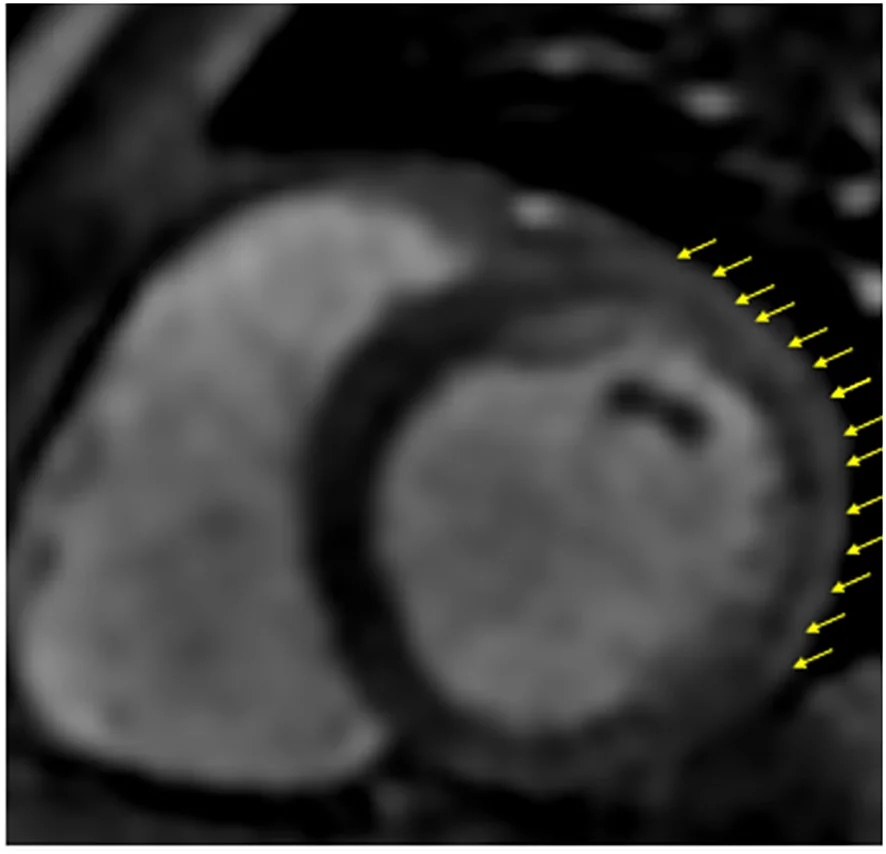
Cardiac MRI performed two weeks after the second cycle of atezolizumab-bevacizumab (June 2024) showing subepicardial late gadolinium enhancement (arrows) involving the lateral wall of the left ventricle, consistent with acute immune checkpoint inhibitor-associated myocarditis.
